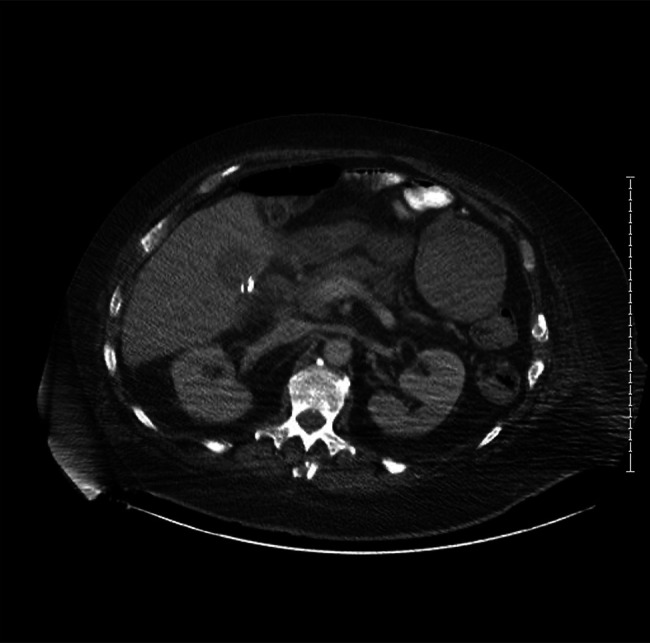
Axial computed tomography image shows a nonspecific fluid collection in the gallbladder fossa and subtle haziness anterior to the pancreatic head.
